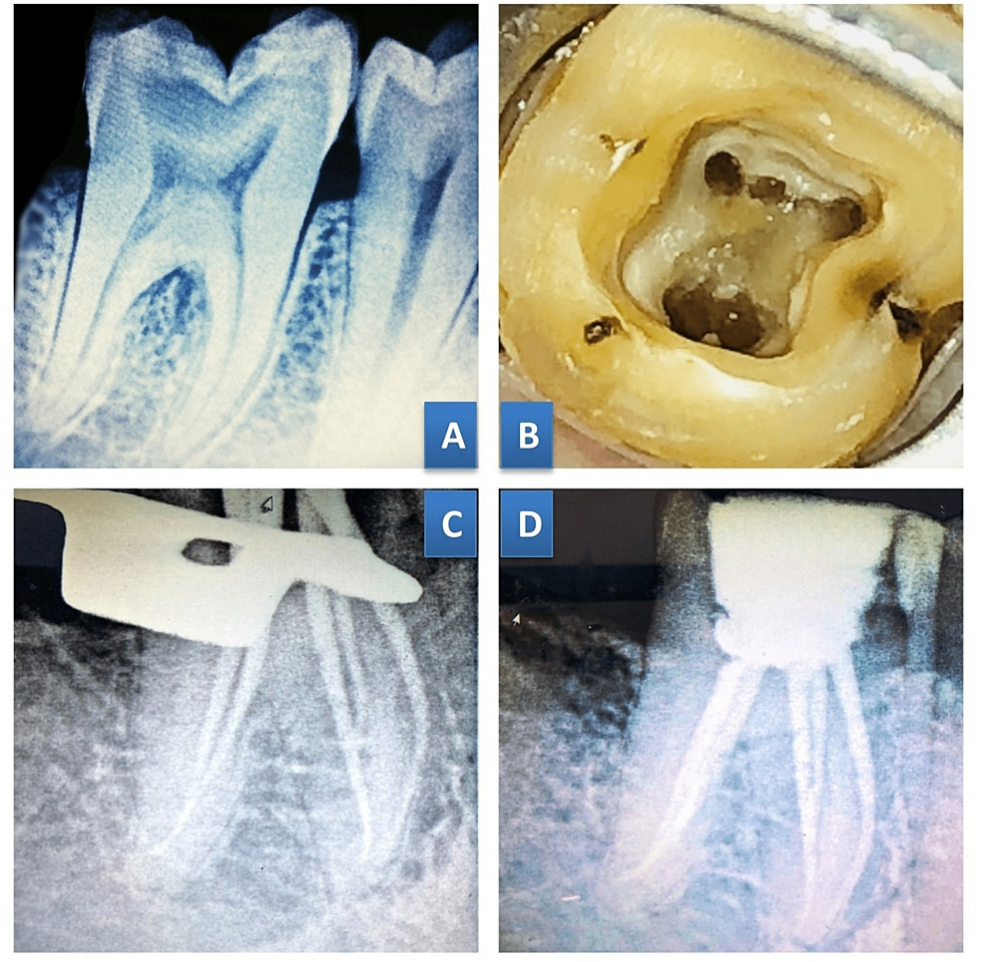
Case 1. Middle mesial canal merged with mesiobuccal canal at the middle third region in 36 (A) Preoperative radiograph;  (B) Access cavity preparation; (C) Master cone radiograph; (D) Post-obturation radiograph
